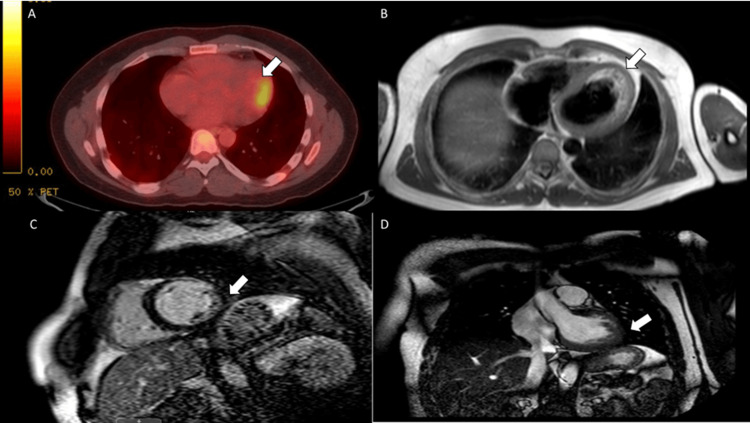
F-18 FDG metabolic imaging A: A small area of the mid-anterolateral myocardial wall increased metabolic activity suggesting focal myocardial inflammation. B: T2 sequences demonstrate focal hyperintense signal involving the mid-anterolateral wall. C: Short-axis view showing left ventricular delayed enhancement consistent with scarring/fibrosis involving the transmural mid-anterolateral wall and subendocardial and mid-wall distal anterolateral and mid and distal anterior wall segment. D: Long-axis view. FDG: Fluorodeoxyglucose
